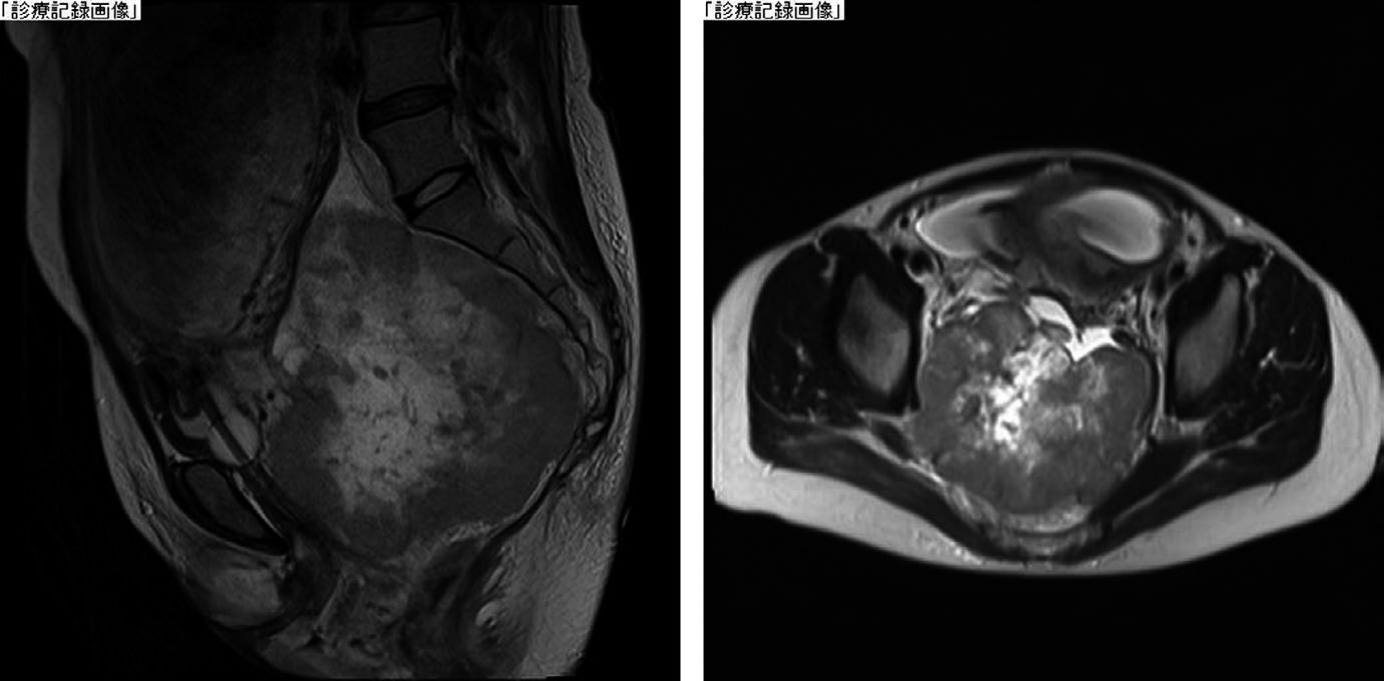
MRI image showed an 11 × 12 cm solid mass in the pelvis at 25 wks of gestation
